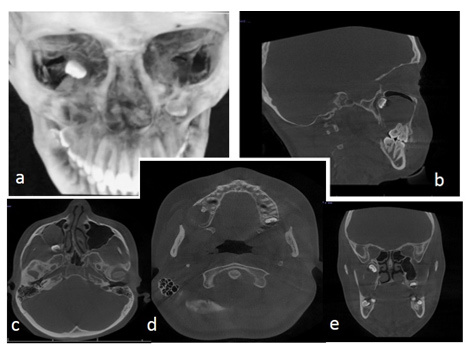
Multiplanar cone beam computed tomography (CBCT) sections: (
